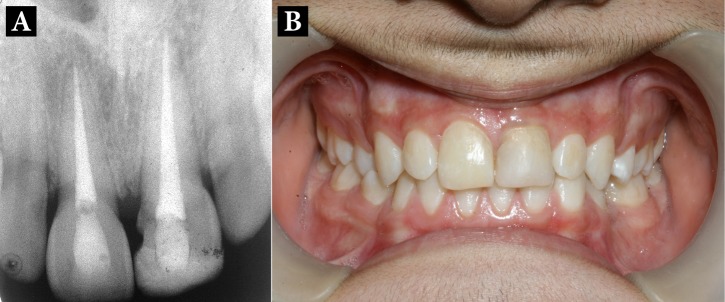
Three-year follow up images; A) Radiographic; B) Intraoral
